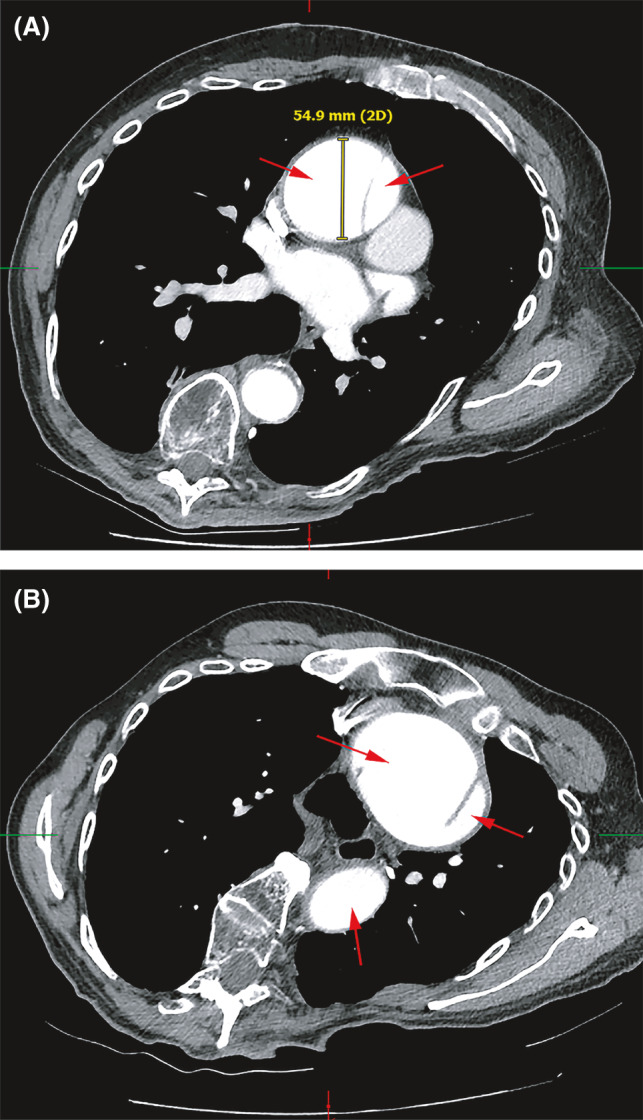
Chest CTA with contrast showing preoperative acute DeBakey type I dissection. (A) Ascending aortic dissection with true and false lumens visualized. Proximal ascending aorta with aneurysmal dilation at 54.9 mm. (B) Descending thoracic aortic dissection with true and false lumens identified. Previous thoracic aorta synthetic dacron graft visualized and intact
